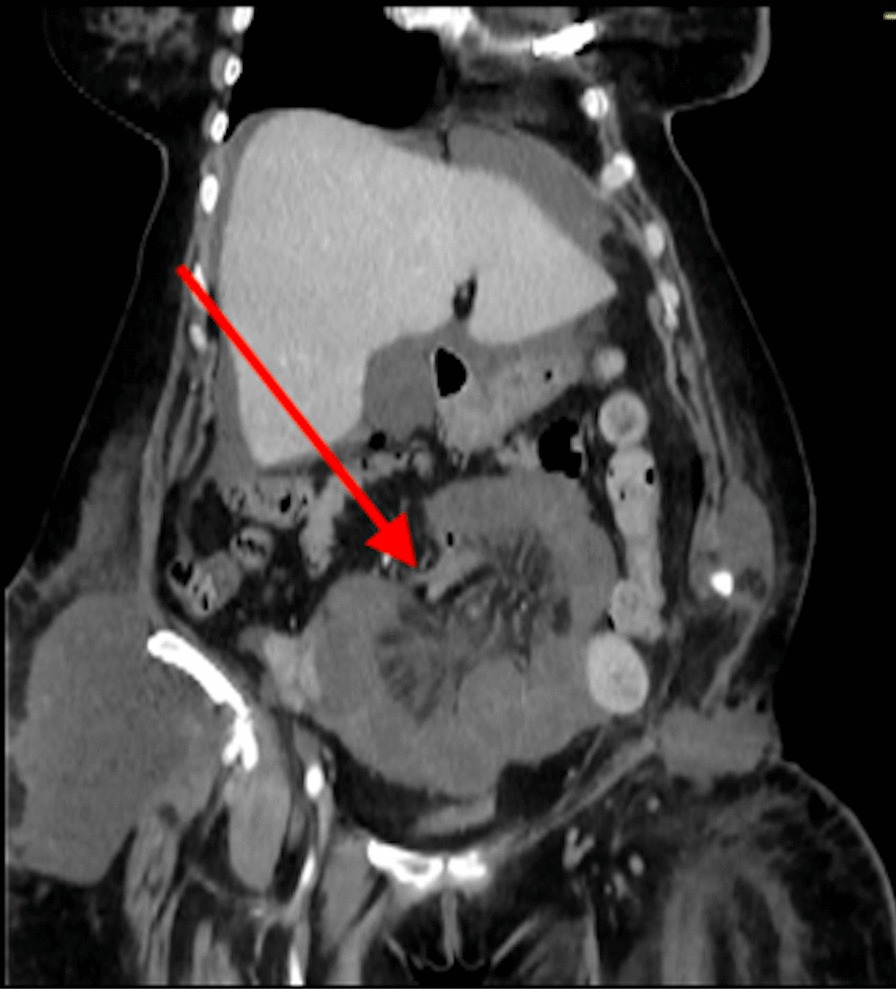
Abdominal CT scan: coronal views. Internal hernia and beginning of bowel ischemia (arrow)
